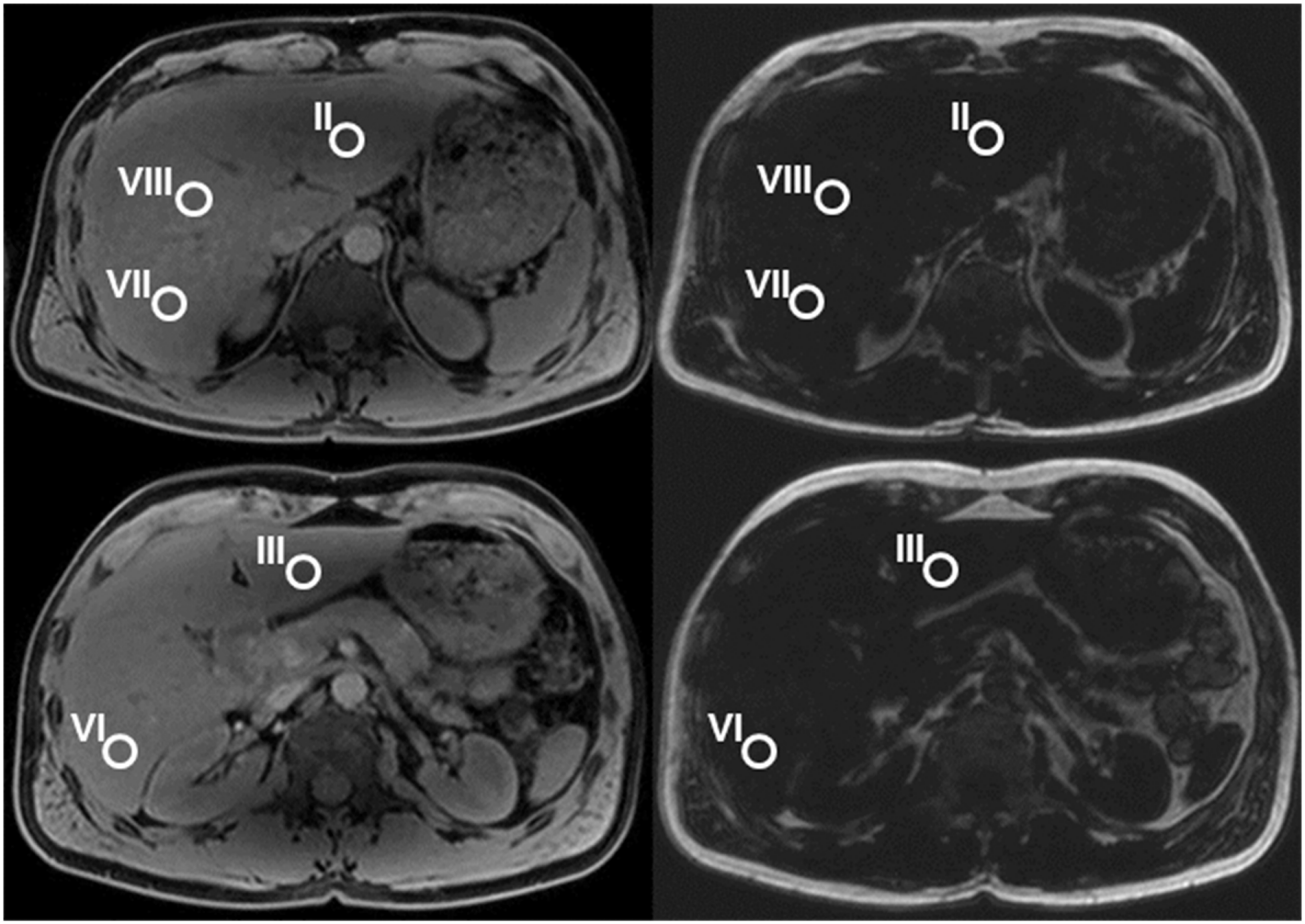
Axial water-signal-only (left side) and fat-signal-only (right side) MR images in a 31-year-old woman. Regions of interest (ROIs) were placed in liver segments II, III, VI, VII and VIII on the water-signal-only images and copied to the fat-signal-only images.
